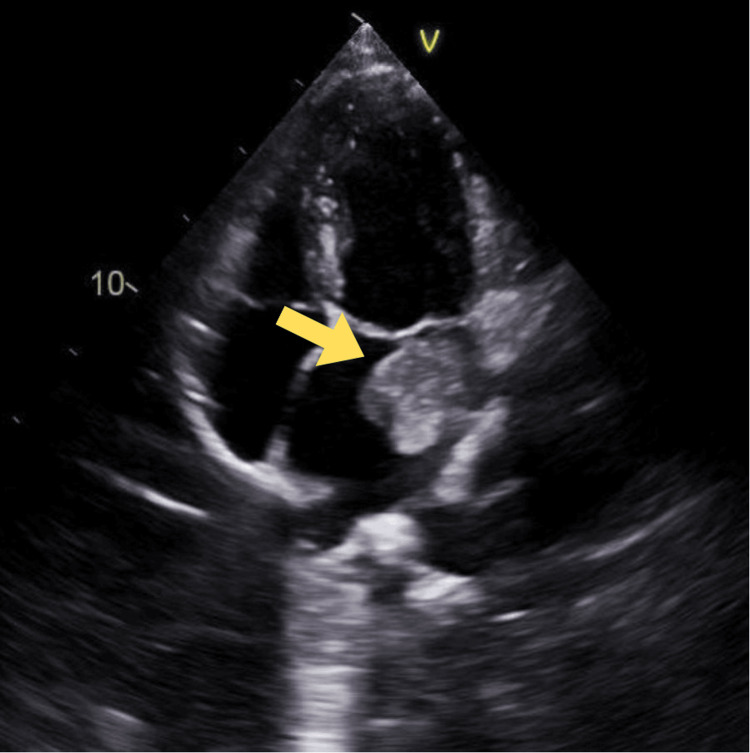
Transthoracic echocardiogram showing a moderately dilated left atrium with a large, irregular 8.3 cm mobile mass in the superior left atrial cavity
